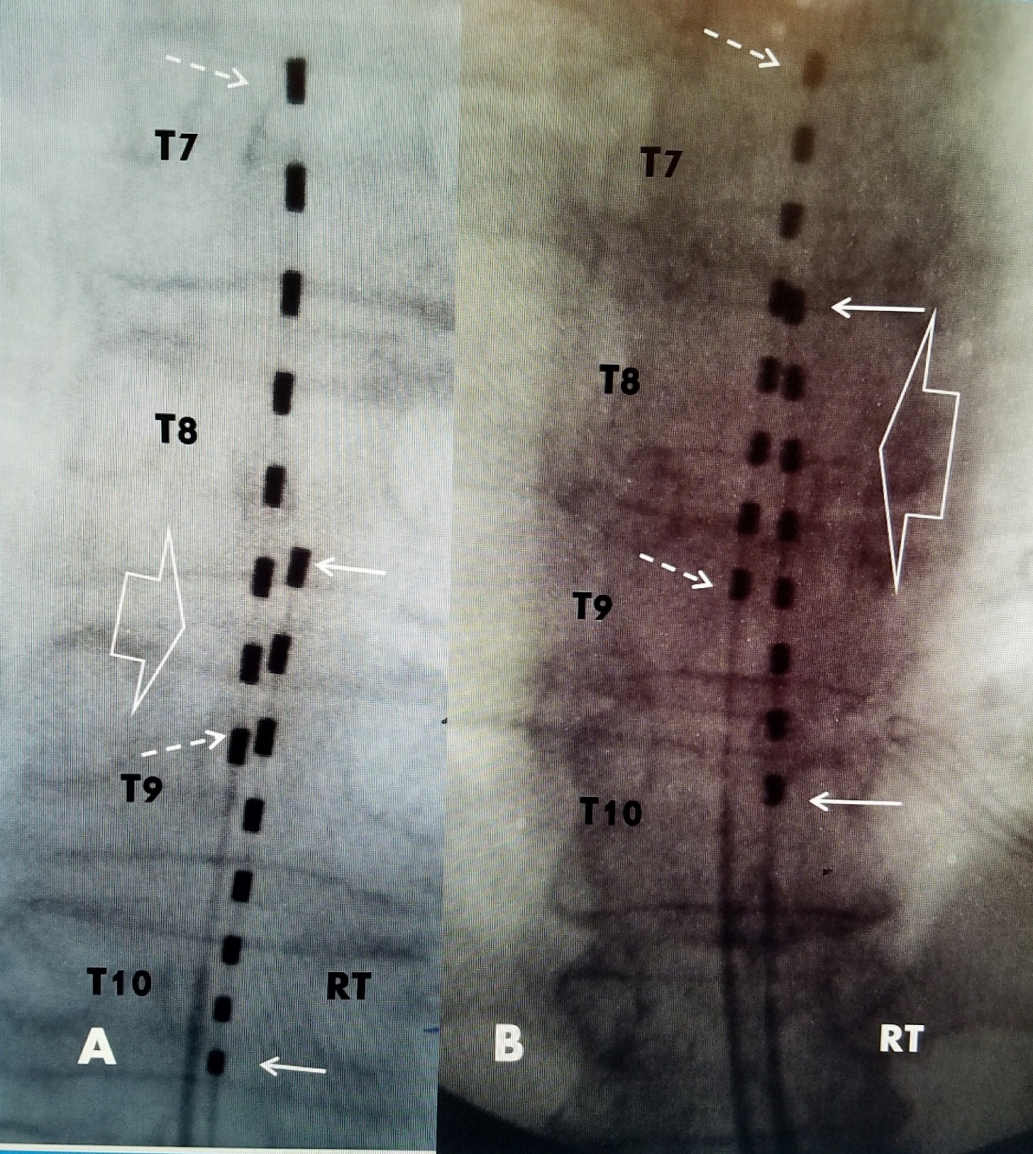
Examples of using overlapping percutaneous leads to get a wider stimulation area. A: Patient with failed back surgery syndrome (FBSS) and painful diabetic peripheral neuropathy affecting both feet. Anterior posterior radiograph showing midline lead extending superiorly from upper T7 to mid T9 (dashed white arrows) and the 2nd midline slightly right lead covers lower T8 thru mid T10 (white arrow). The overlapping three electrodes between T8/9 (open white arrow) allowed bipolar stimulation to the lumbar spine. All leads are centered towards the midline. The second lead from T8/9 to T10/11 provided lower extremity coverage for the peripheral neuropathy. B: Patient with FBSS and right chronic knee pain after joint replacement: Radiograph showing lead positioned in the midline from upper T7 to mid T9 (dashed white arrow) for the lumbar pain. The right lead is parallel from the top T8 to upper T10 (solid white arrow) but more to the right side that provided coverage for the right knee. There is a broad area of five electrodes that overlap from T8 to mid T9 allowing bipolar cross stimulation (open white arrow) for his lumbar pain.
